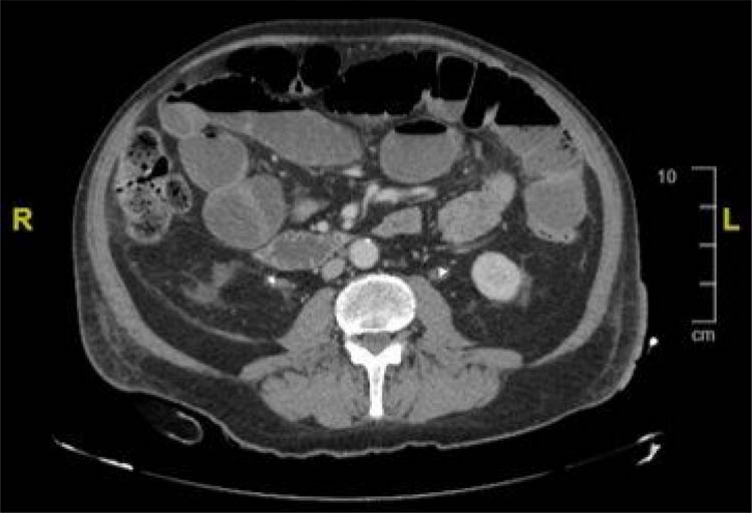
CT image showing distended bowel loops indicating bowel obstruction caused by the pelvic mass.
